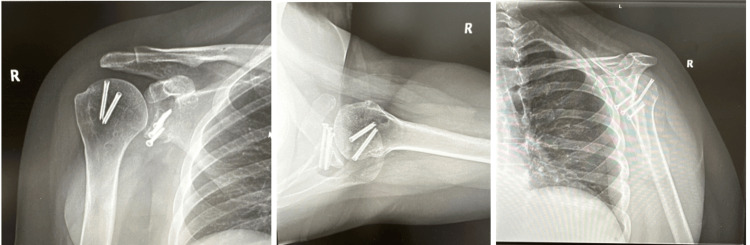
Anteroposterior (AP), axillary, and scapular Y radiographic views (left to right) at 12 months after right shoulder iliac crest autograft glenoid fixation with proximal humerus osteochondral bone wedge allograft procedure.
